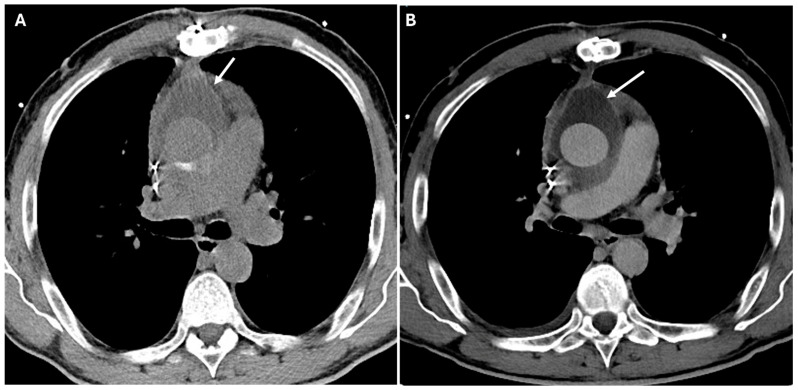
CT images of infection of perigraft fluid collections. Panels (A,B) show a seroma with slightly thickened and hyperemic walls near the aortic graft (white arrows) after a Bentall procedure.
